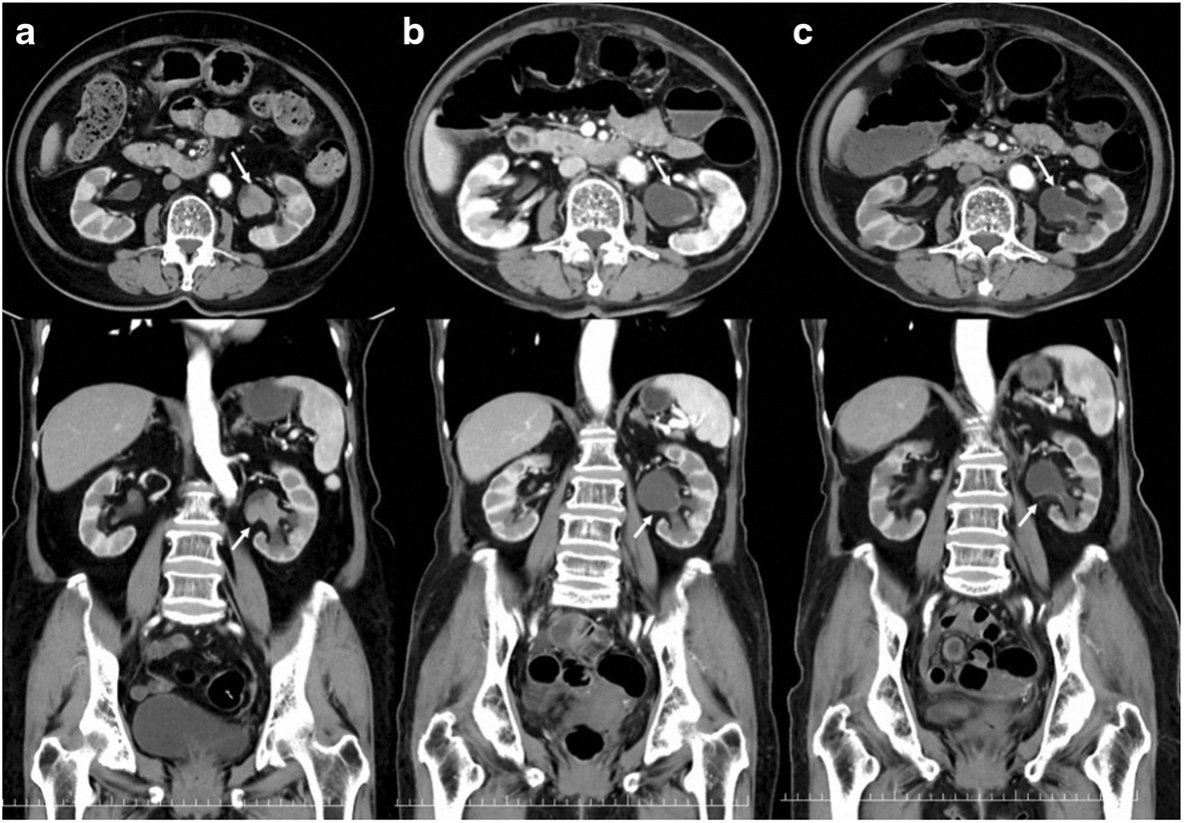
Repeated contrast-enhanced computed tomography (a–c) shows that the left pelvic tumor, which showed no mutation (wild type), had shrunk (*white arrow*). The three pictures were taken over the course of the treatment
